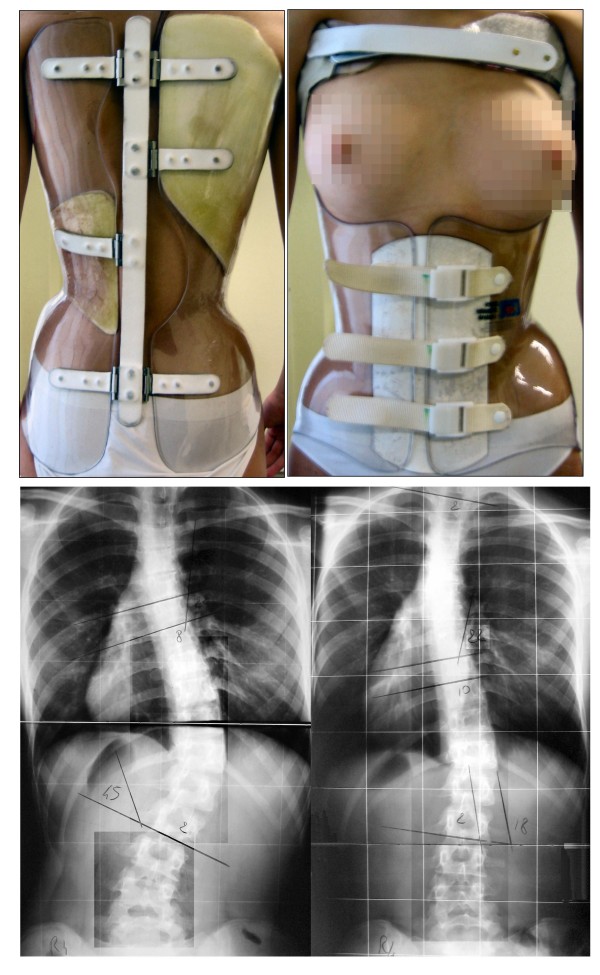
The Sforzesco brace. The Sforzesco brace for a thoracic right lumbar left curve, in the antero-posterior and postero-anterior views. An example of out brace x-rays results after six months is reported.
